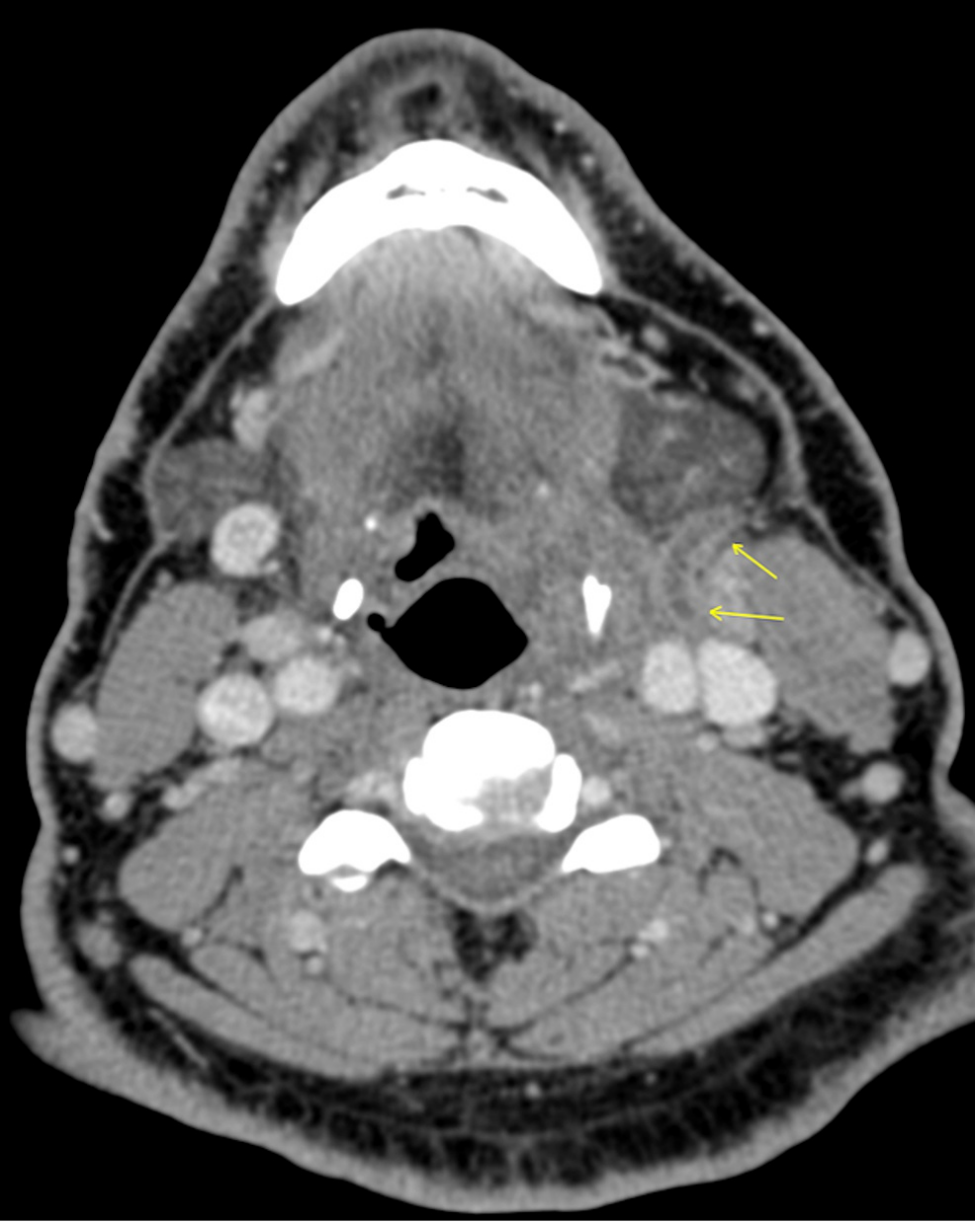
CT soft tissue neck with contrast showing with left facial vein thrombosis (yellow arrow)
